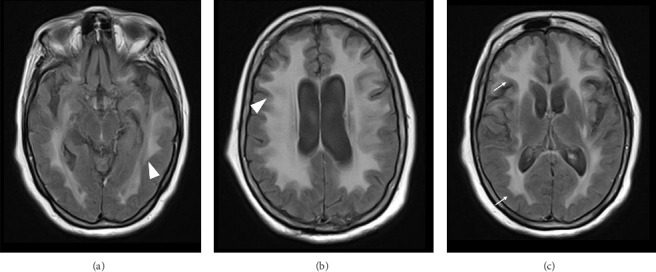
MRI of the patient's brain in the T2/FLAIR sequence, generalized white matter involvement is observed. (a) A slice at the midbrain level shows white matter involvement in the temporal lobes. (b) The section at the lateral ventricles level shows generalized hyperintensity with extension into the overlying cortex. (c) A slice at the level of the insula and thalamus shows white matter involvement in the frontal and parietal lobes.
